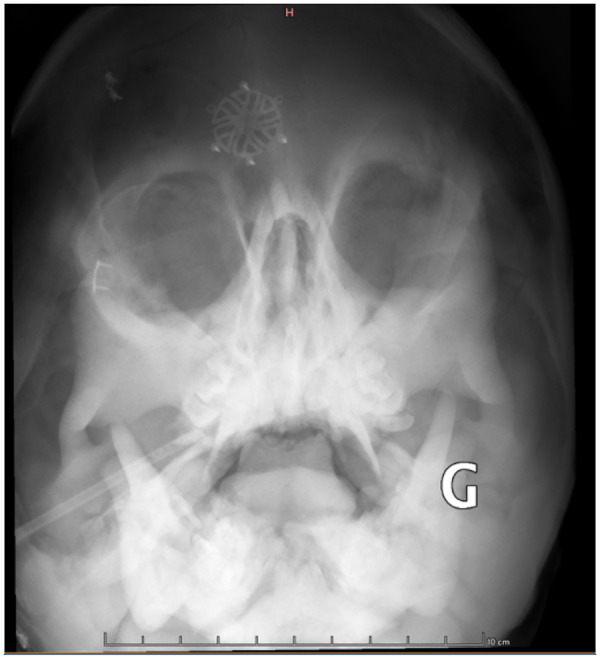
Blondeau projection radiograph of the skull of a 10-year-old child with OP, showing typical skull base thickening.
